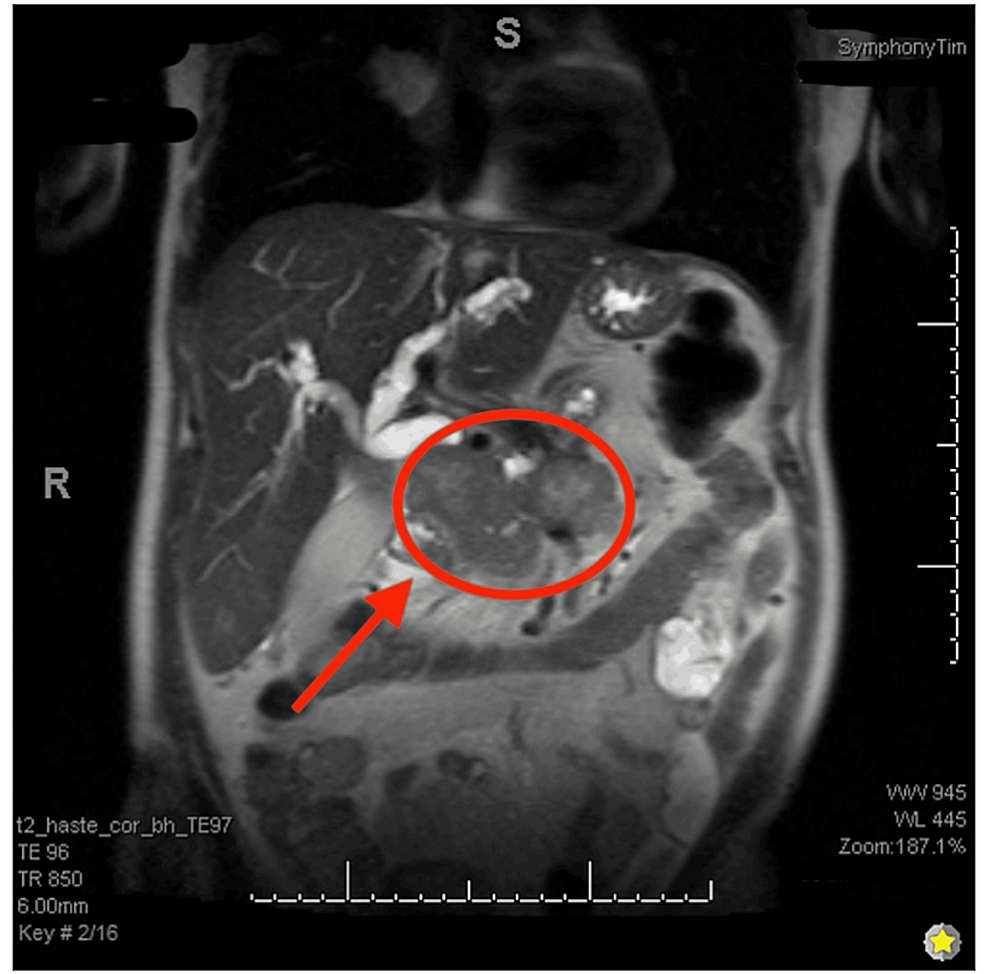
T2-weighted MRI abdomen with and without MRCP (coronal view) showing pancreatic mass post-gadolinium contrast administration. A pancreatic mass of 8.2 x 9.6 x 6.6 cm with dilation of intra and extrahepatic bile ducts. MRCP: magnetic resonance cholangiopancreatography
